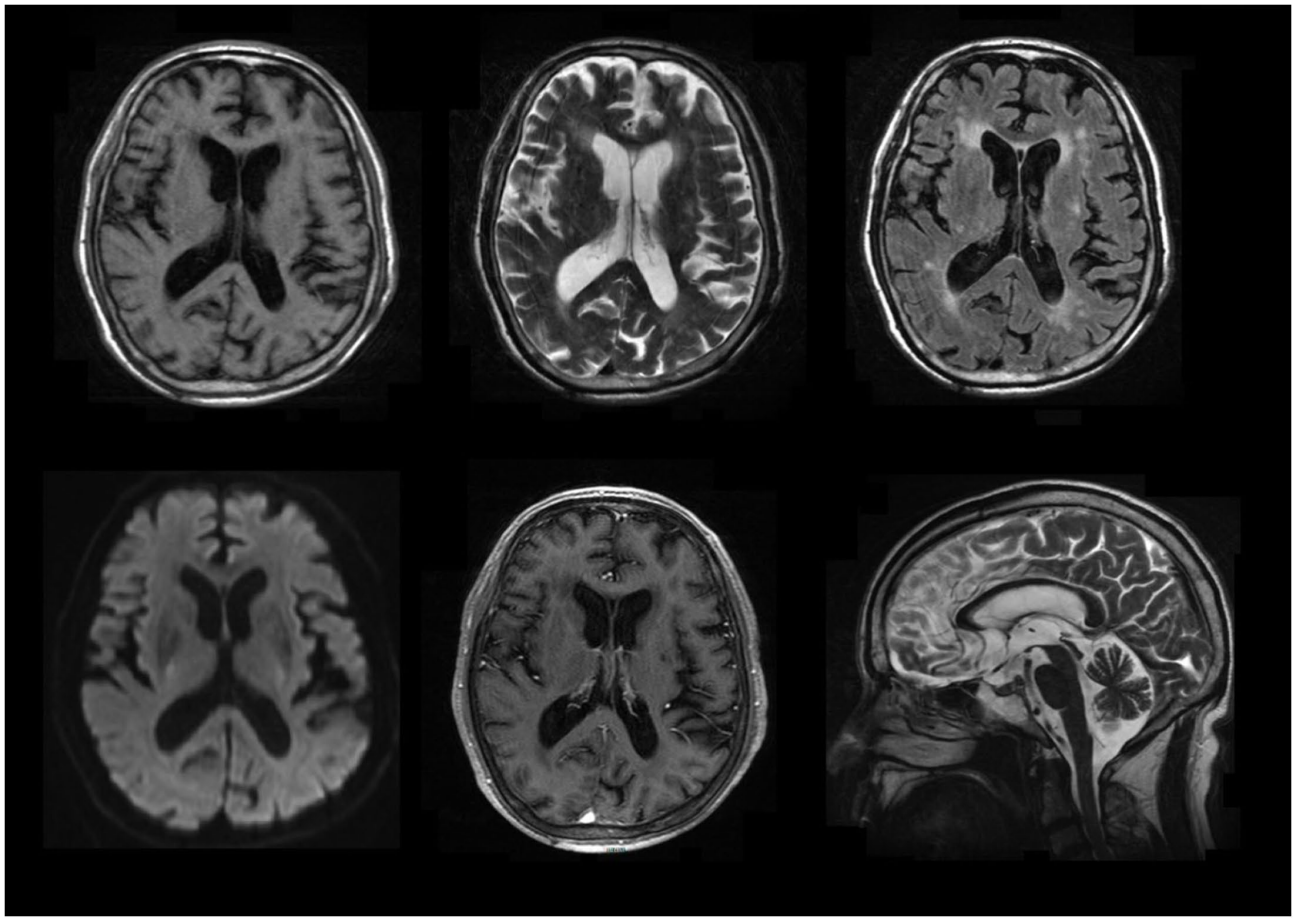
Selected brain MR images. It showed ischemia within the periventricular area and centrum semiovale but revealed no contrast-enhanced lesions.
